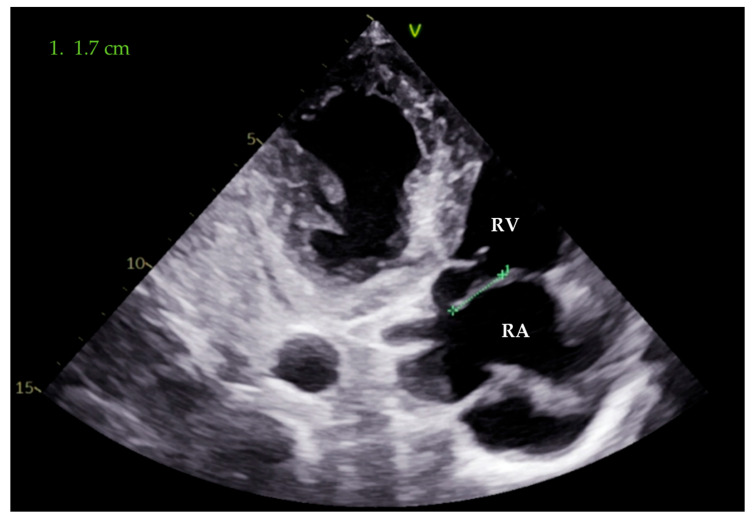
Transthoracic image (apical four chamber view, right ventricle focused). The length of the vegetation attached to the anterior leaflet of the tricuspid valve is 1.7 cm (green line). RA—right atrium; RV—right ventricle.
